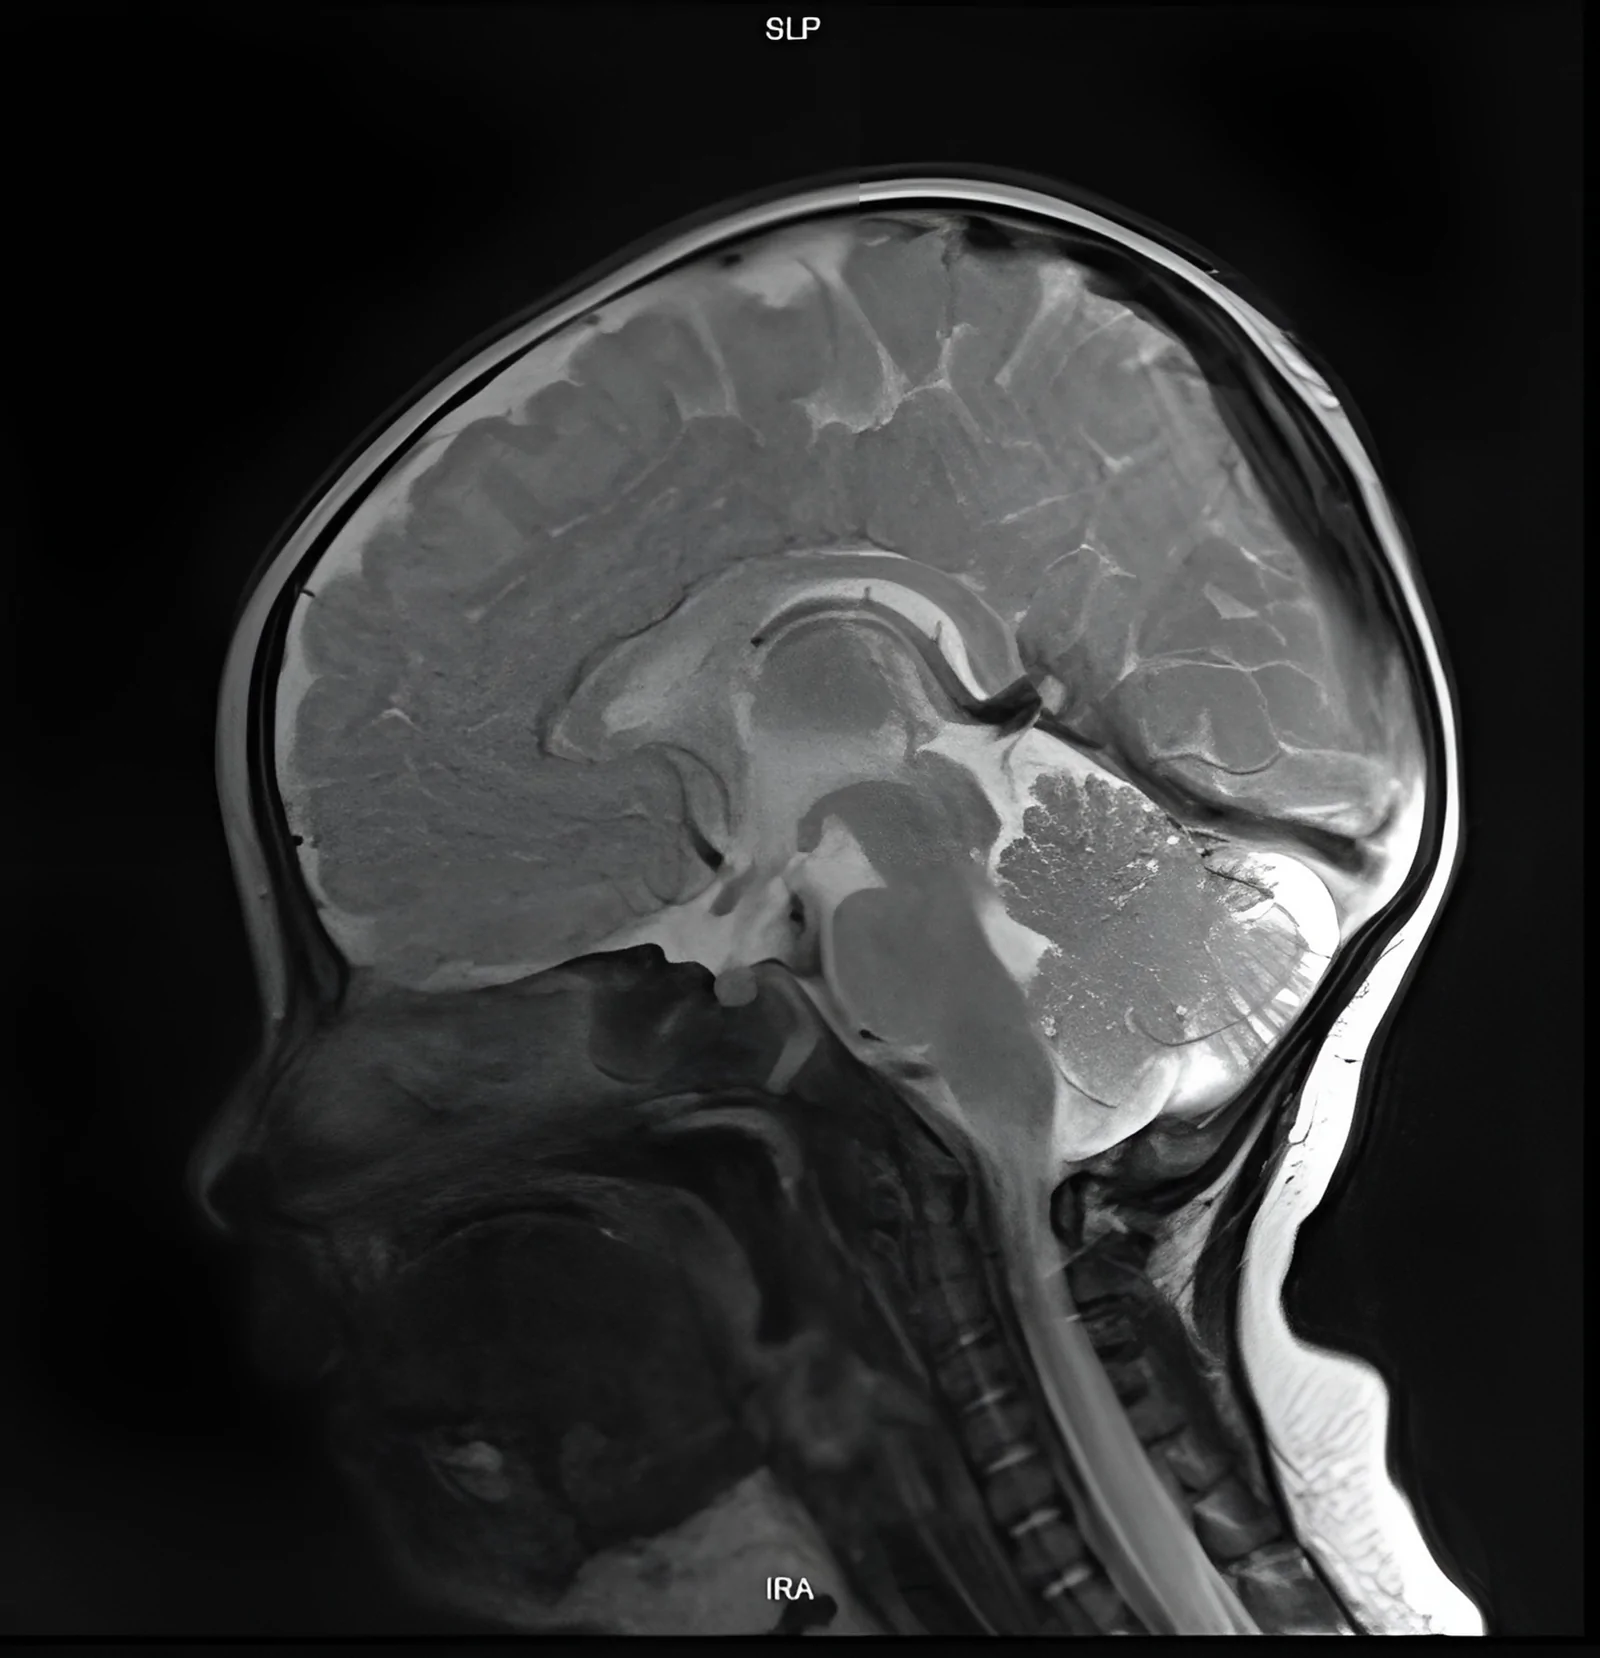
Brain magnetic resonance imaging (MRI) demonstrates normal intracranial anatomy with no evidence of structural abnormalities
